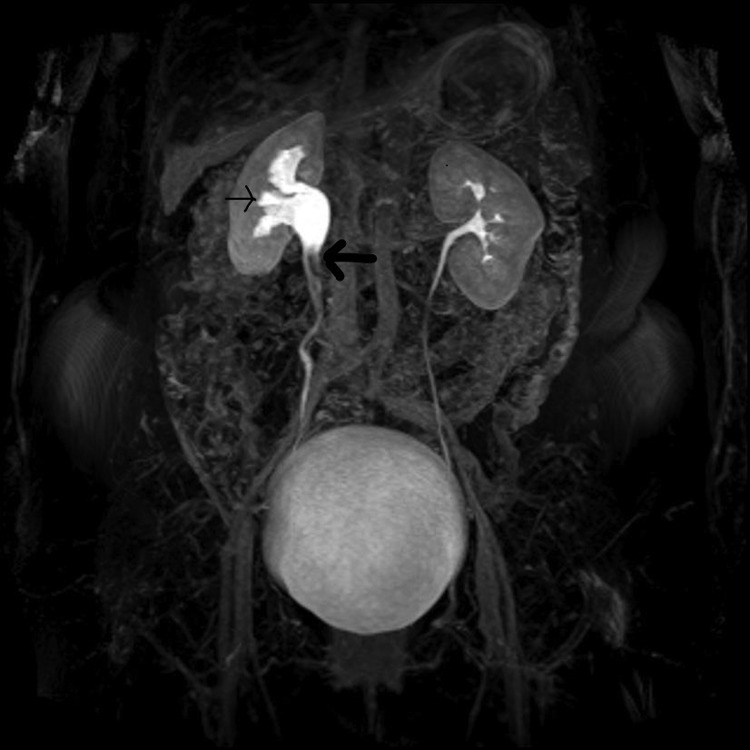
Three-dimensional MR urography with T2-weighted images; filling defects in right proximal ureter (*bold arrow*), causing ureteral obstruction and moderate pyelocaliceal upstream dilation (*thin arrow*).
